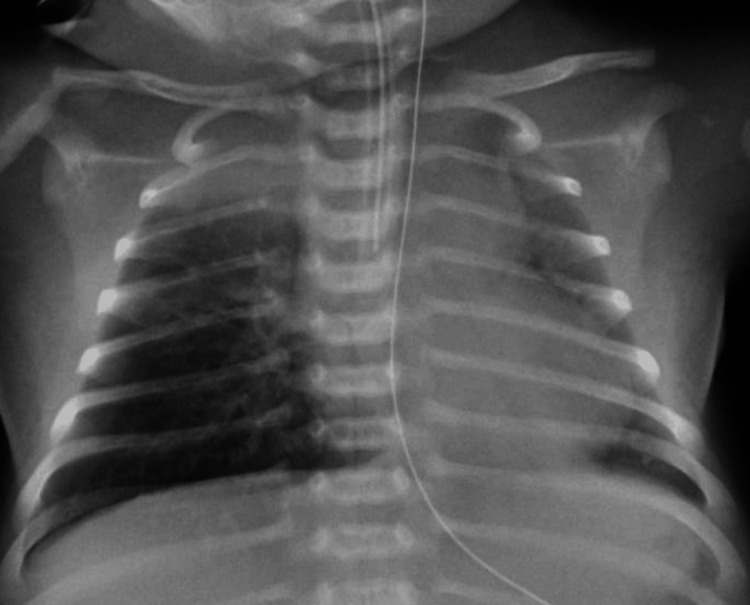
Chest radiograph on hospital day 2 showing infiltrative shadows in the right upper lung field and hilar region.
